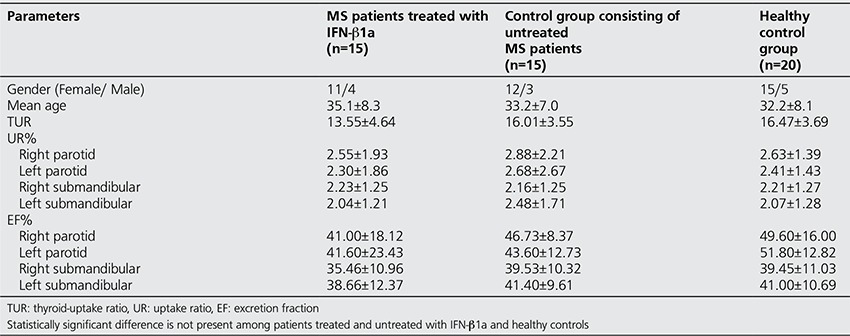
Comparison of quantitative scintigraphic parameters of salivary glands in MS patients treated with IFN-β1a, untreated MS patients and healthy controls
